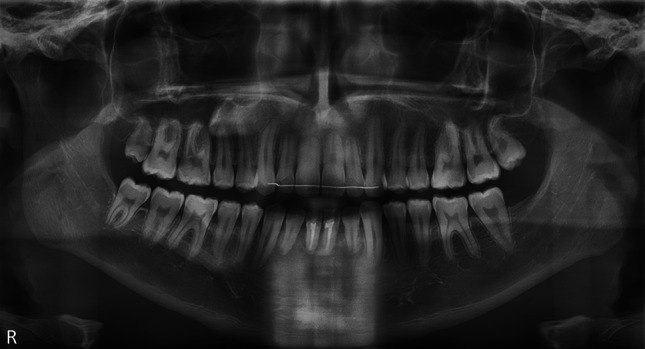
Panoramic radiography of a mandible with insufficient IANC corticalization
